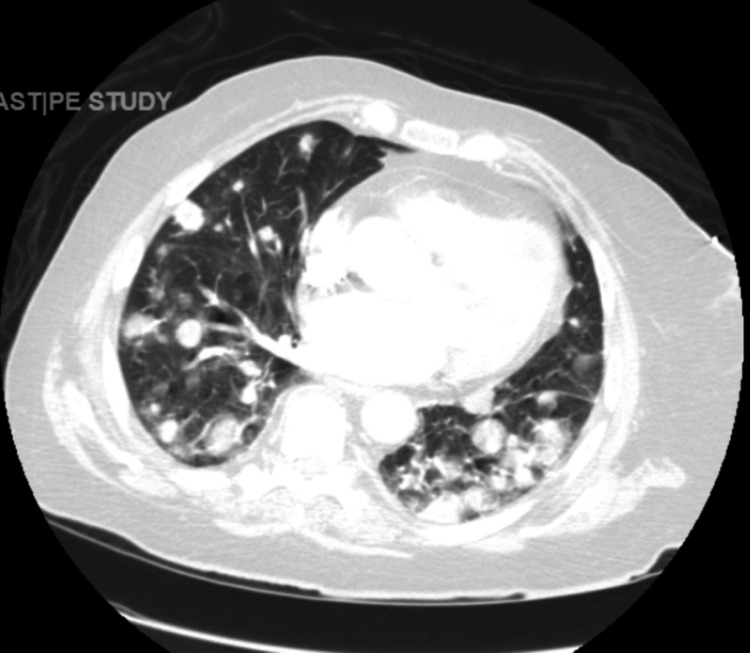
Computed tomographic presentation of pulmonary nodules with bilateral pulmonary edema
